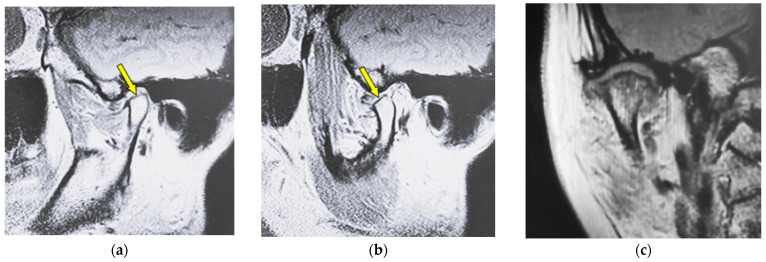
MR image (proton-density-weighted) of a 51-year-old woman (disc displacement without reduction, right disc): (a) sagittal oblique cross-section imaging in the mouth-closing position; (b) sagittal oblique cross-section imaging in the mouth-opening position; (c) coronal oblique cross-section imaging in the mouth-closing position. The arrow shows the part with flattening.
